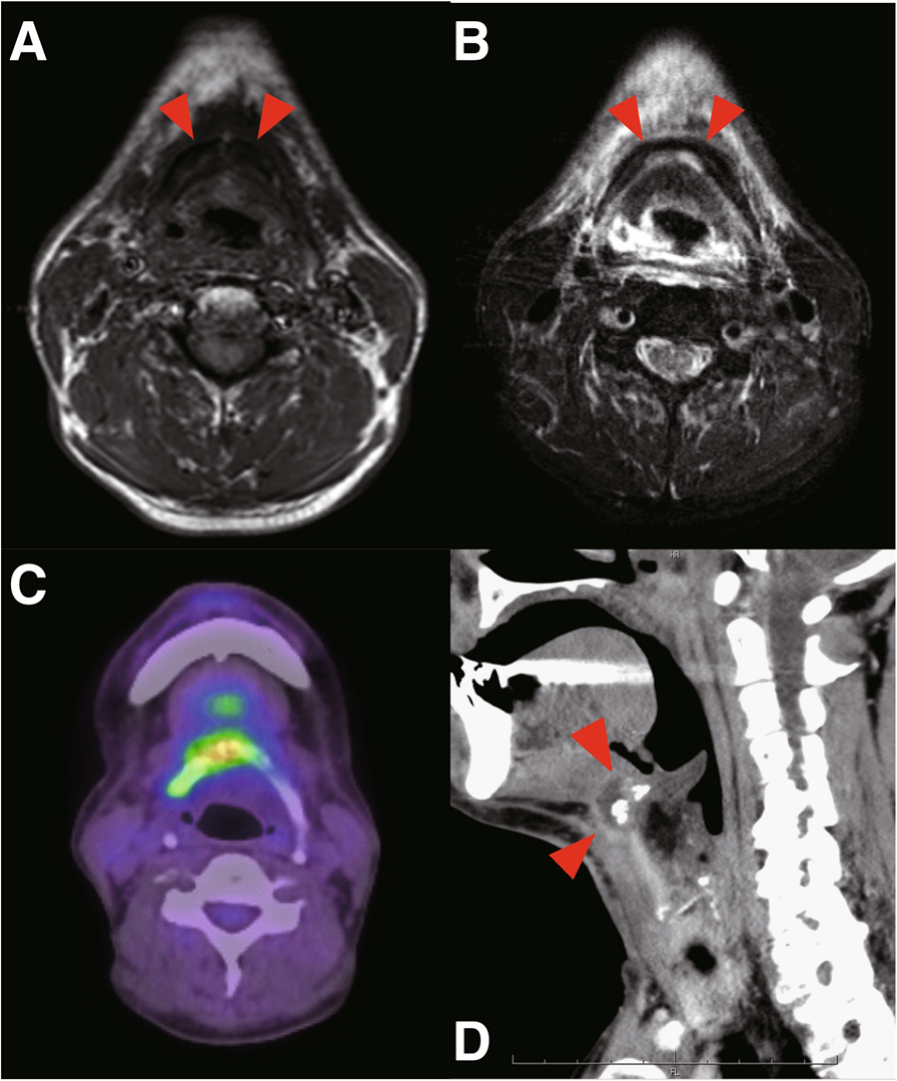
a Signal intensity partially decreased in the hyoid on T1WI, b Mixed high and low signal intensity on T2WI The arrows indicate the ORN of hyoid bone. c The PET-CT image: High FDG activity is observed in the hyoid. d Sagittal view of the CT scan image in the larynx. The *red arrows* indicate disruption of the hyoid with abscess formation
